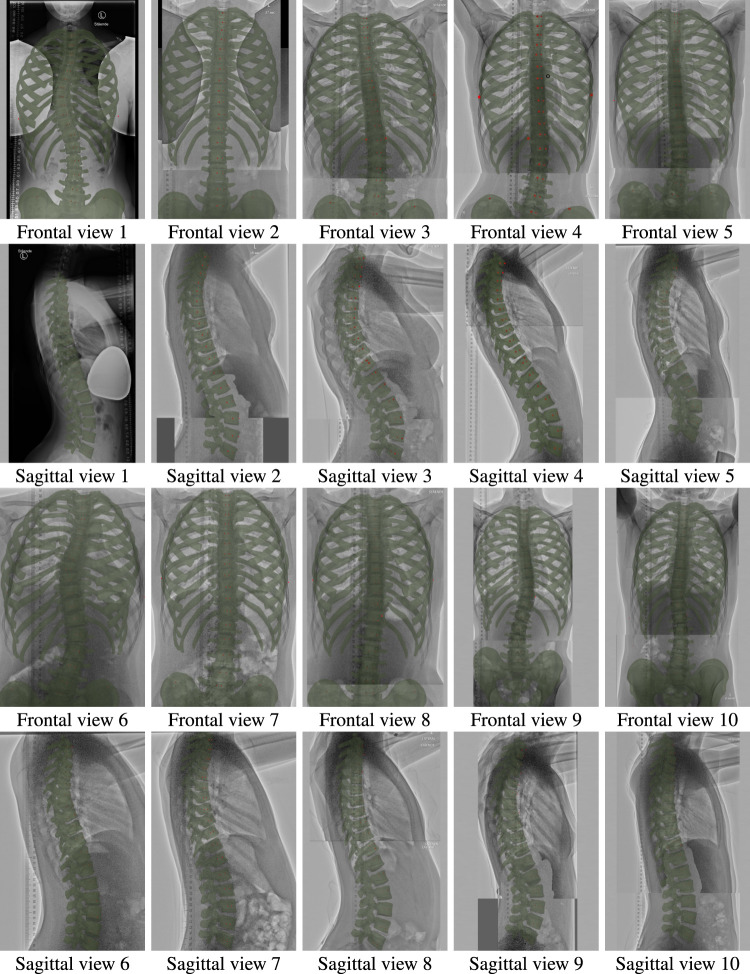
Frontal and sagittal views of all ten patients of the study, with the morphed models overlaid on the input radiographs.
